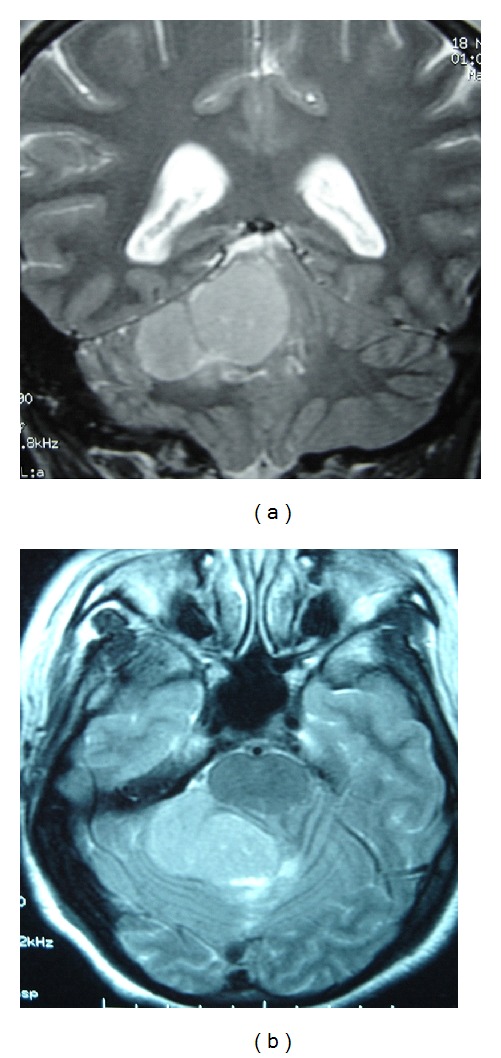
MR showing in T2-weighted hypersignal right cerebellar lesions, with a broad tentorial attachment.
